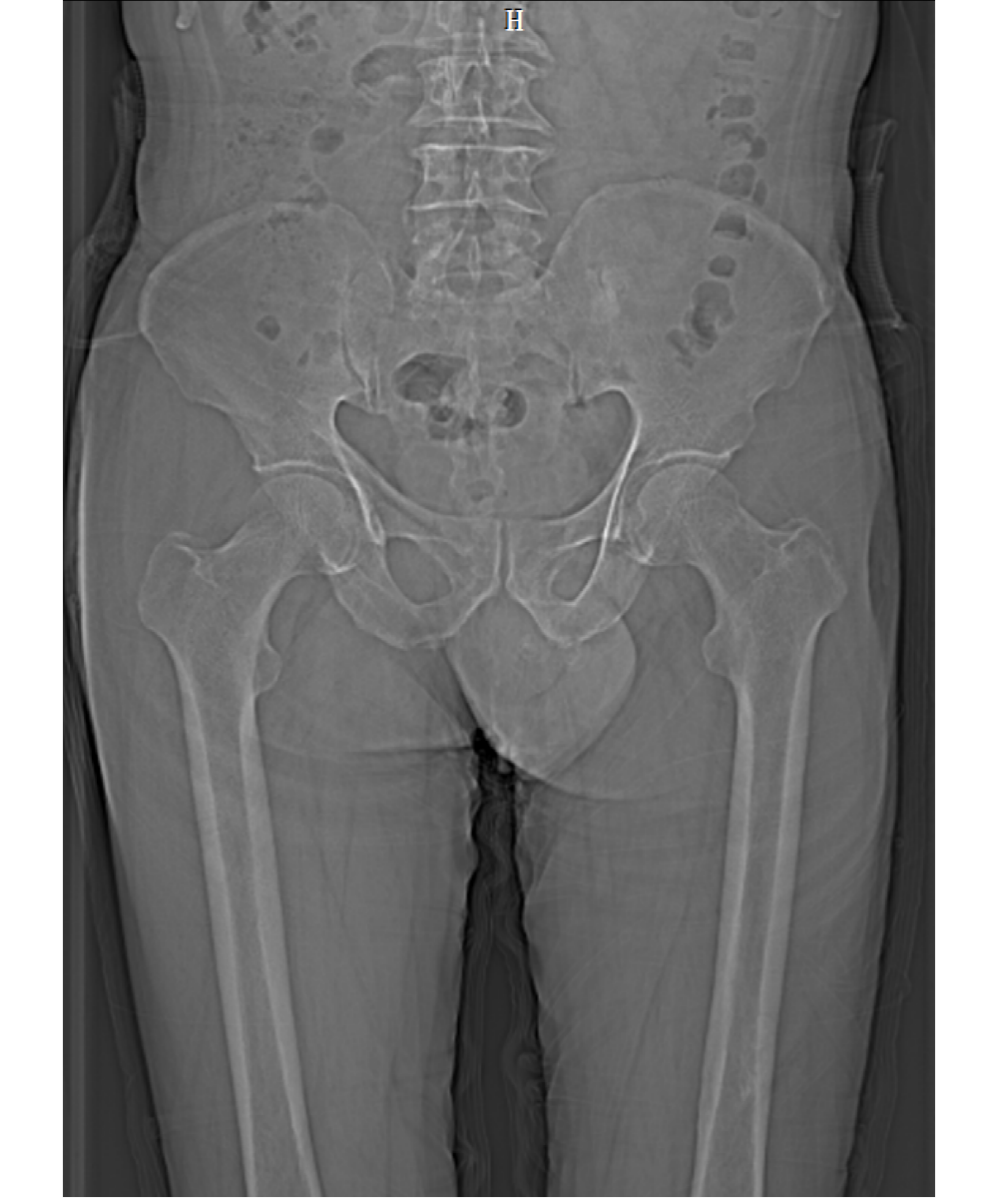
Magnetic resonance image of the older male participant. H: head.
